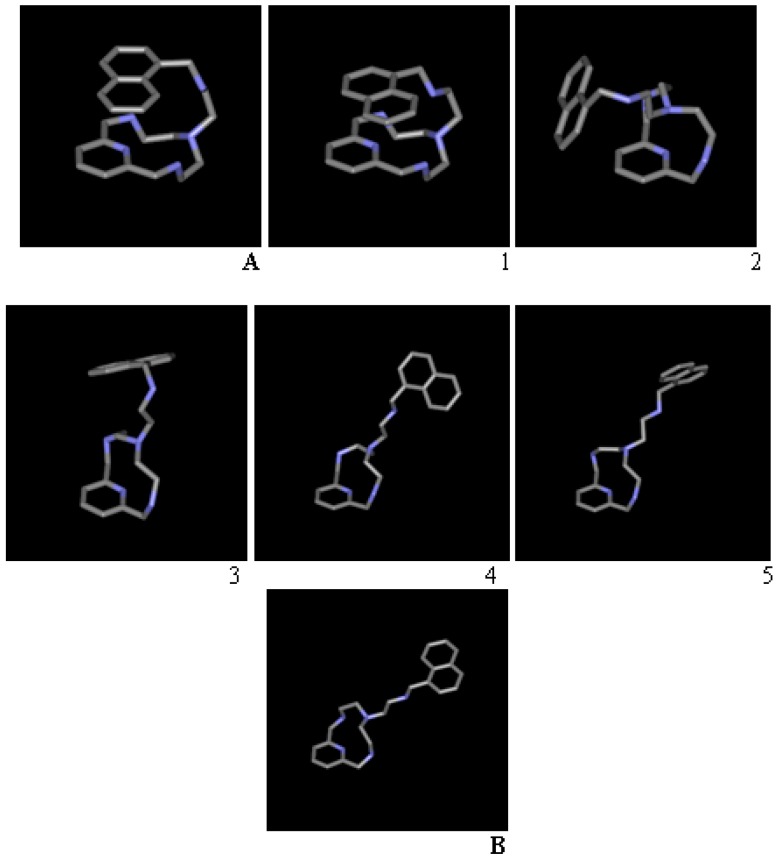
(A,B) Conformations from X-ray minimized in the periodic box. 1–5, conformations obtained from MCMM in vacuum and further minimization in standard water density-constant TI3P box until their respective local minima, for different runs.
